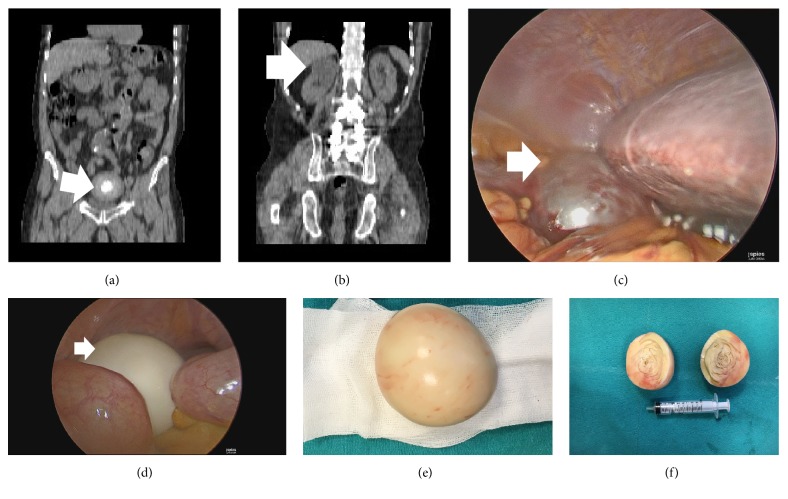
The calcific mass floating independently in abdomen and right kidney mass were diagnosed. (a) Computed tomography revealed a calcific mass with 7 cm diameter that was making pressure on bladder. (b) There was right kidney cyst with 5.3 cm diameter on the upper pole in computed tomography scan. (c) The kidney cyst was decorticated during laparoscopic surgery. (d) The free mass was observed simultaneously in laparoscopic surgery. (e) The mass was taken out directly; there was no attachment around other tissues. (f) The mass was divided into half; it was very rigid.
